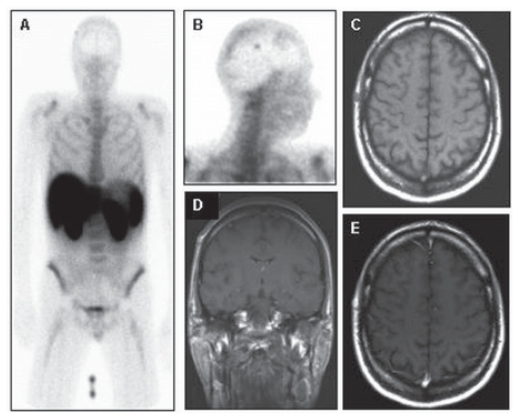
Planar anterior (A) and lateral (B) Annexin V images show focal tracer uptake in the skull right. This lesion correlates with an oval subcutaneous mass with intermediate signal intensity on T1 weighted MR image and homogeneous enhancement on coronal (D) and axial gadolinium-enhanced T1-weighted MR images, identified as a capillary hemangioma.
